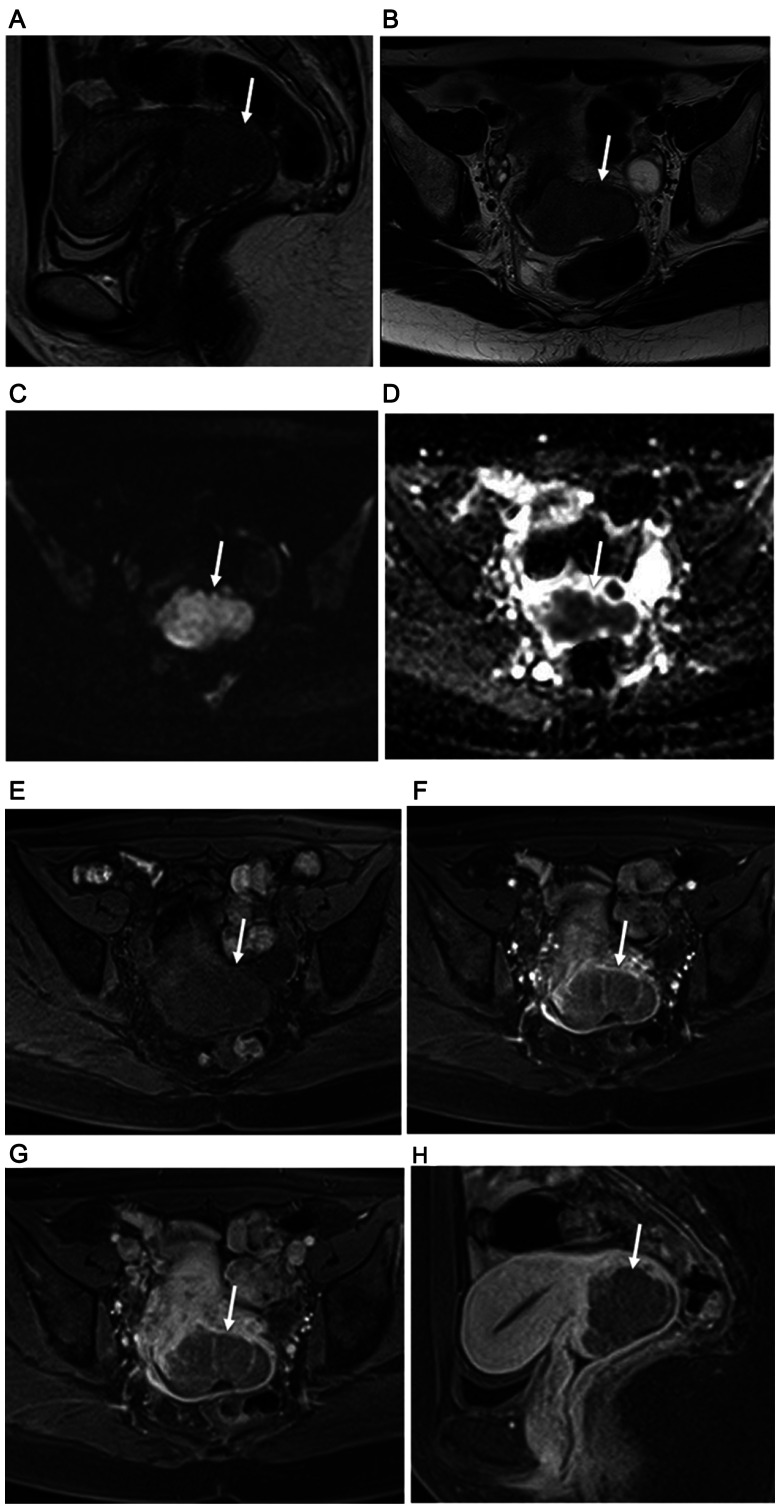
34-year-old woman with FIGO stage IB2 uterine cervical large cell neuroendocrine carcinoma. (A) Sagittal and (B) axial T2-weighted imagings show homogeneous slightly hyperintense signal from large lobulated mass in uterine cervix (arrow). Subsequent surgery confirmed MRI staging of no invasion of vagina and parametrium. (C) Cervical mass with remarkably high signal intensity throughout tumor shown by axial DWI (arrow). (D) Obvious diffusion restriction throughout cervical tumor shown by axial ADC mapping (arrow). Results of dynamic contrast-enhanced axial fat-suppressed T1-weighted imaging found at (E) pre-contrast stage, and (F) 30 and (G) 120 seconds after administration of Gd-DTPA show a weak but gradual enhancement pattern (arrows). (H) Weak tumor enhancement (arrow) shown by sagittal late-phase enhanced fat-suppressed T1-weighted imaging (240 seconds after Gd-DTPA administration). Recurrence was noted 10.3 months after surgery and death at 20.5 months.
